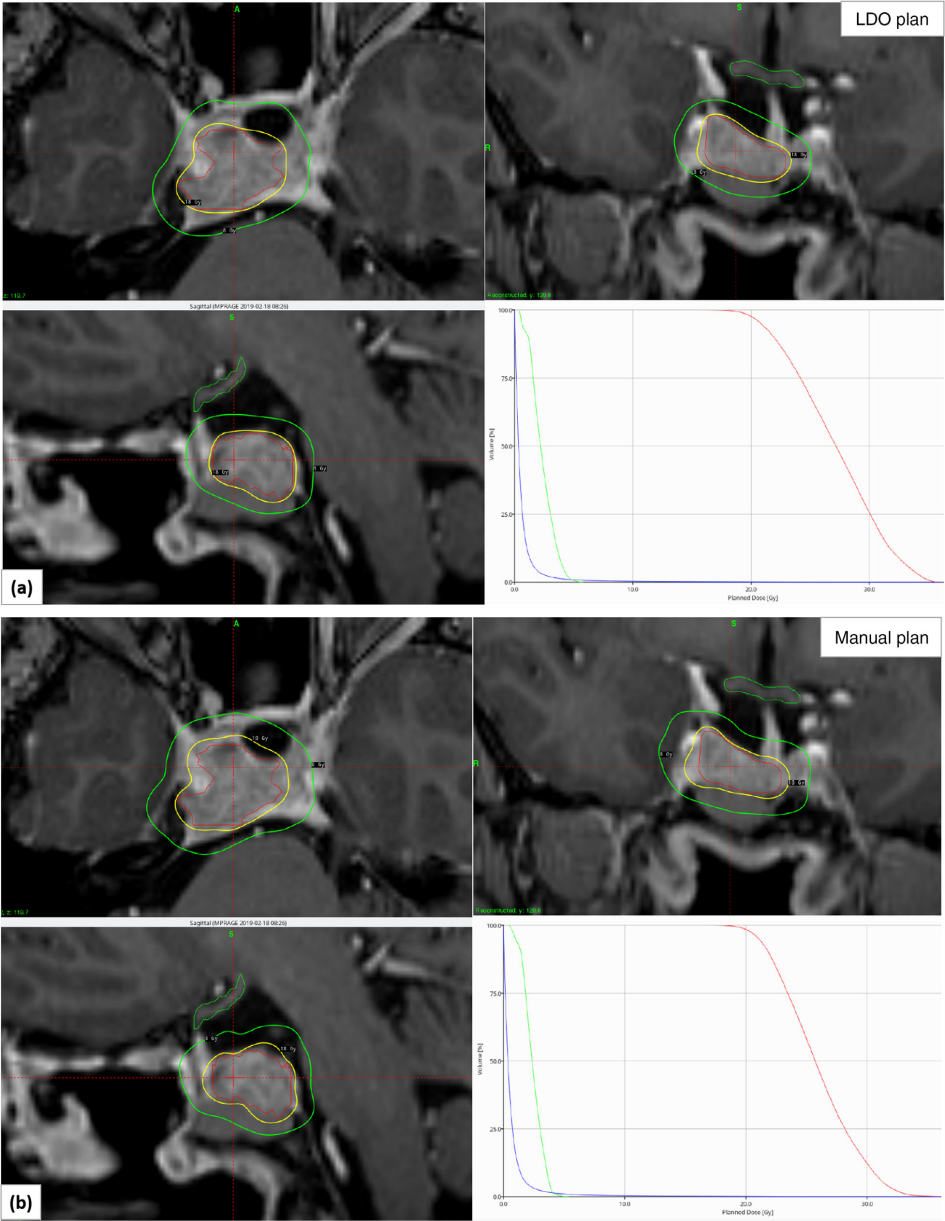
Axial, coronal, and sagittal views of isodose distribution (yellow, 18 Gy prescription and green, 8 Gy isodose lines) in the post‐contrast MPRAGE MRI images demonstrating the dosimetric differences between the lightning dose optimizer (LDO) (a, upper panel) and manual clinical plan (b, lower panel) of the representative pituitary cancer patient with the major improvement of the plan quality via new fast optimizer. The graphs show the dose–volume histogram (DVH) plot for pituitary adenoma (red), optic apparatus (green), and body contour (blue). Compared to the manual plan, LDO improved conformity and dose gradient and significantly reduced normal brain *V*
